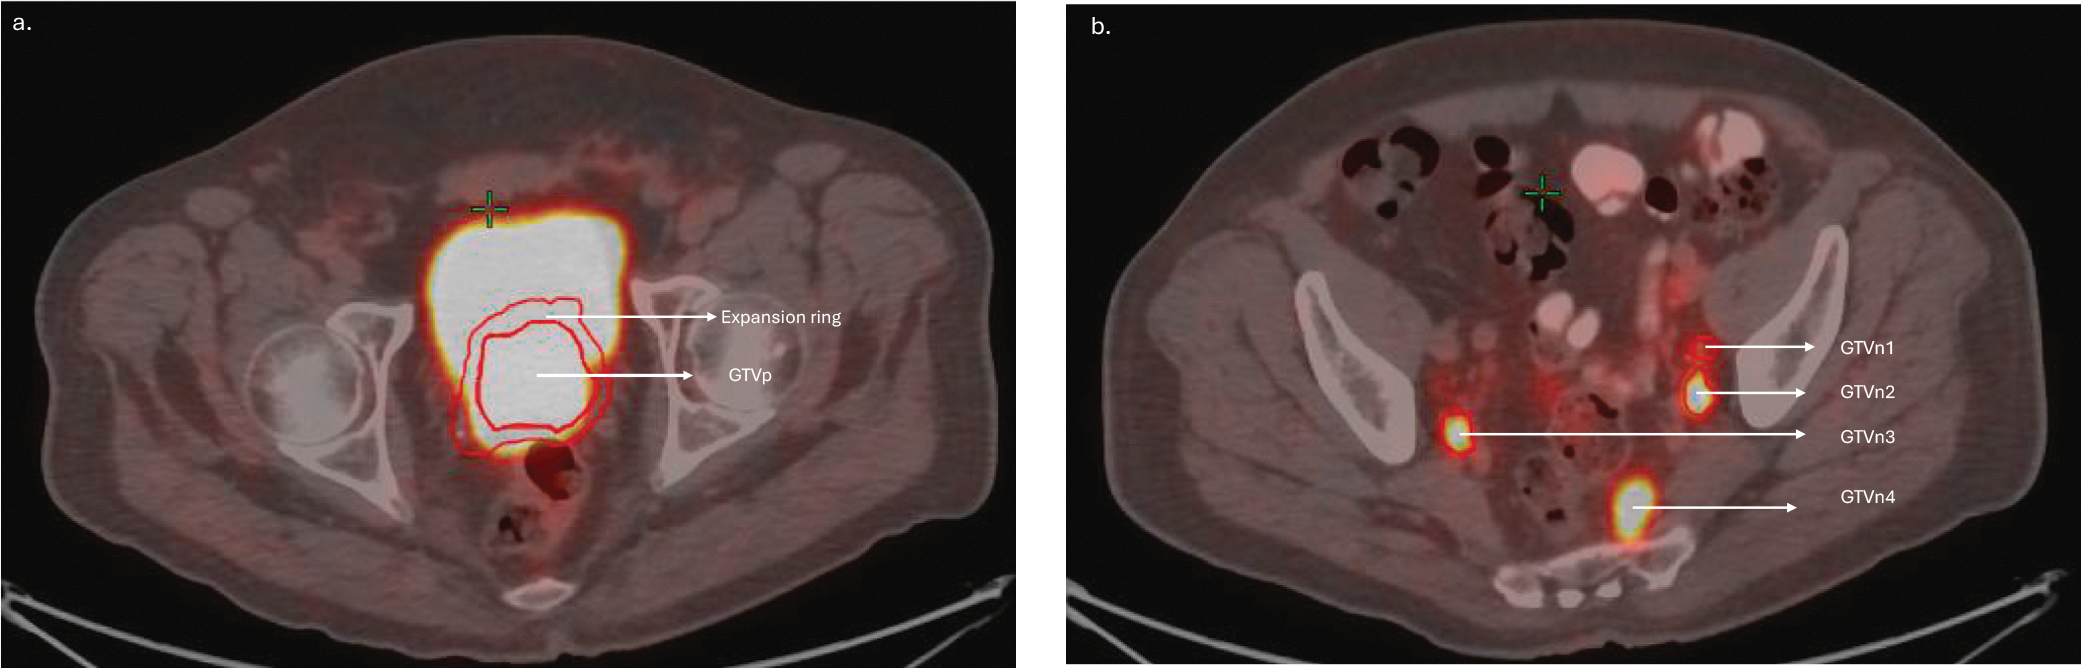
Representative figures showing the regions of interest including (a) the primary prostate tumor volume (GTVp) and expansion ring region and (b) the node regions (GTVn1–n4) on the pre-registered PSMA-PET/CT scan slices of a patient.
